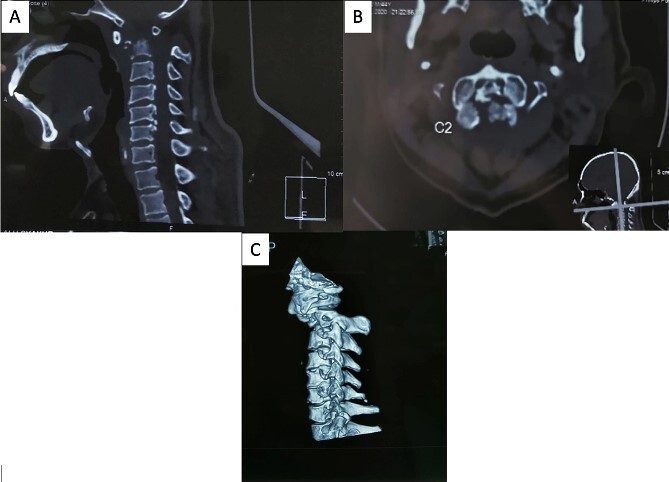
A cervical CT scan revealed an odontoid fracture and severe atlantoaxial dislocation. (A) Sagittal view, (B) axial view, and (C) lateral view of three-dimensional CT revealed atlantoaxial dislocation with odontoid fracture.
